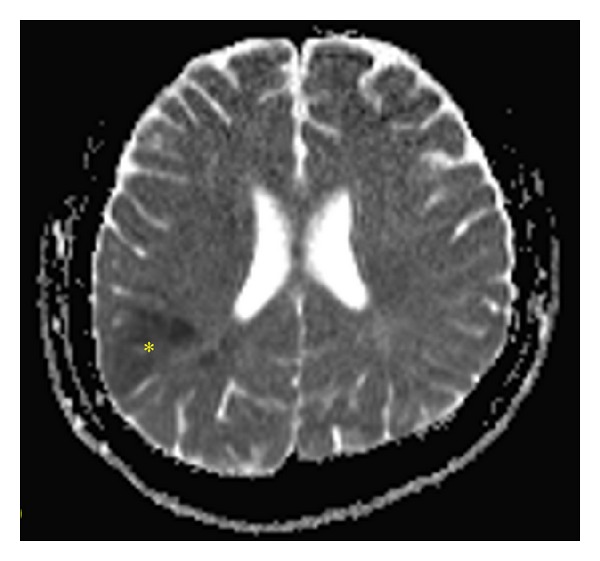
MRI of patient with the supratentorial ischemia as a consequence of microembolisation (paradox embolisation excluded).
